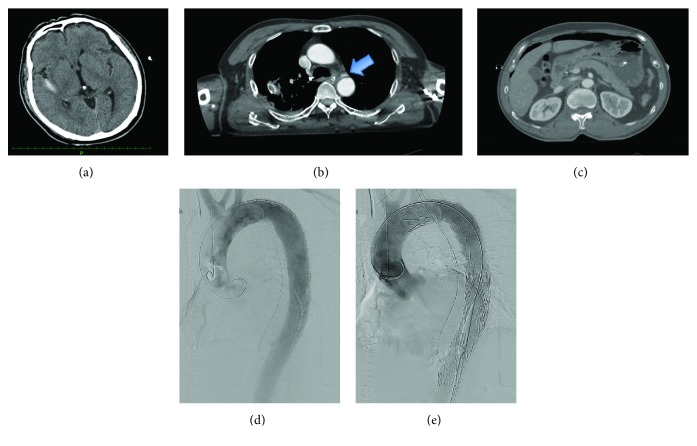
Case 3. Enhanced computed tomography performed in the emergency room after resuscitation: (a) head axial image, subarachnoid hemorrhage was observed; (b) thoracic axial image, pseudoaneurysm was observed in the distal aortic arch (arrow); and (c) abdominal axial image, free air in the abdomen was observed. Intraoperative aortography: (d) before deploying the stent graft and (e) after deploying the stent graft. There were no endoleaks.
